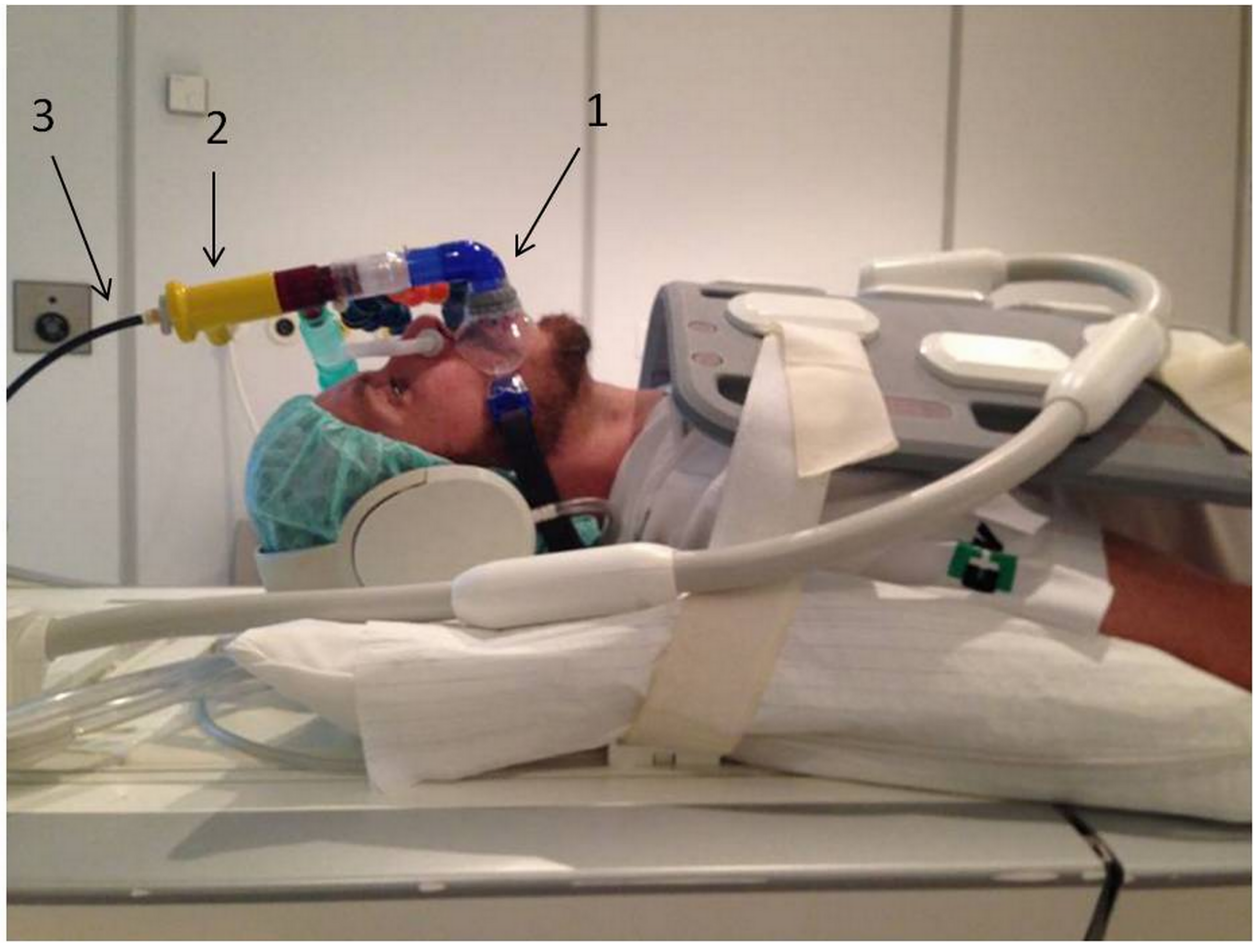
High frequency non invasive ventilation in an awake non-sedated subject lying on a MRI table. The Percussionaire^®^ ventilator remains outside the room and is connected to the non invasive interface (mouthpiece (1)) via the Phasitron (2) using a 6 meter long circuit (3).
